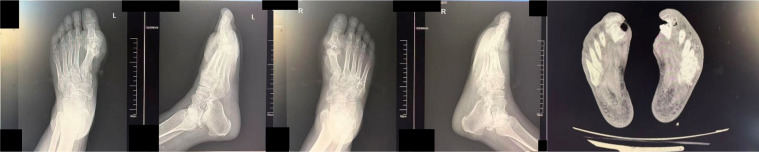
X-ray images of the patient’s bilateral feet.
